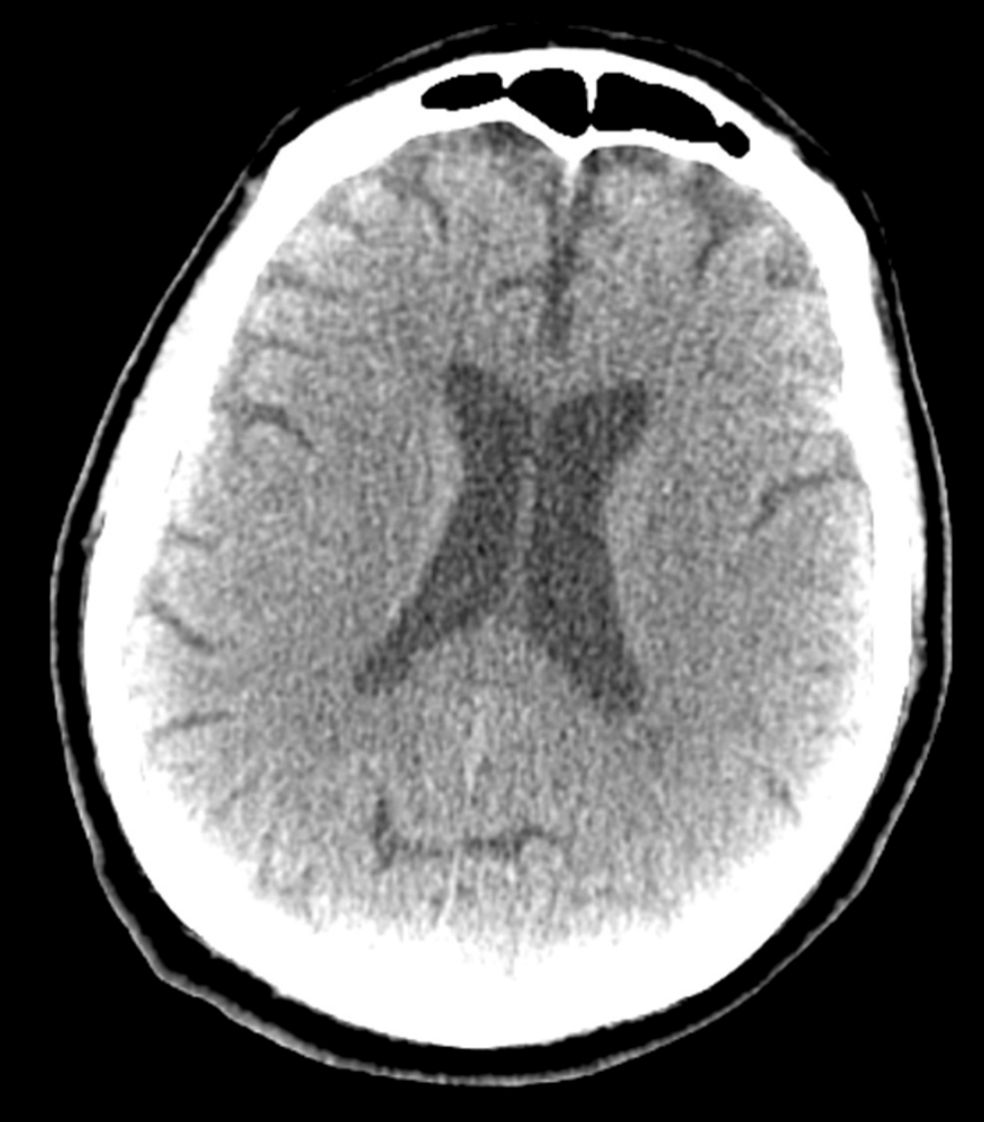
CT brain without contrast shows that the fourth ventricle is midline, and ventricles and subarachnoid space are normal in size. There is no evidence of acute infarct, edema, or hemorrhage.
